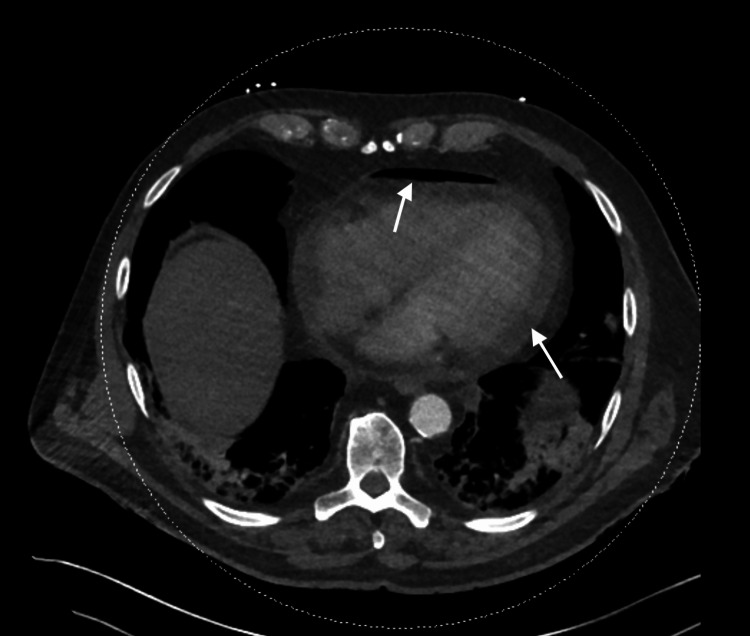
Computed tomography angiography showing pneumopericardium and pericardial effusion
